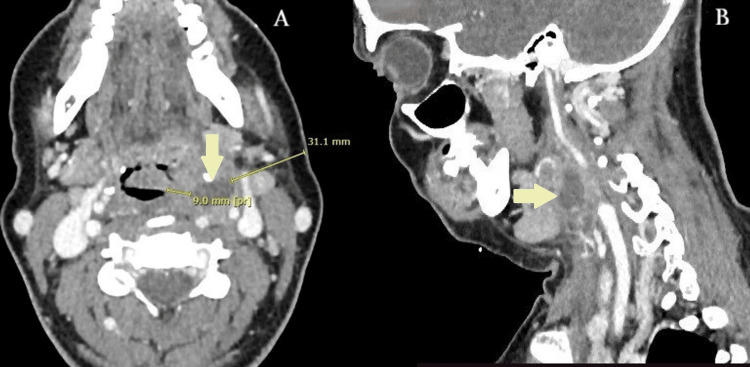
CT scan of the neck demonstrating the parapharyngeal abscess: (A) axial view; (B) sagittal view. CT, Computed tomography
